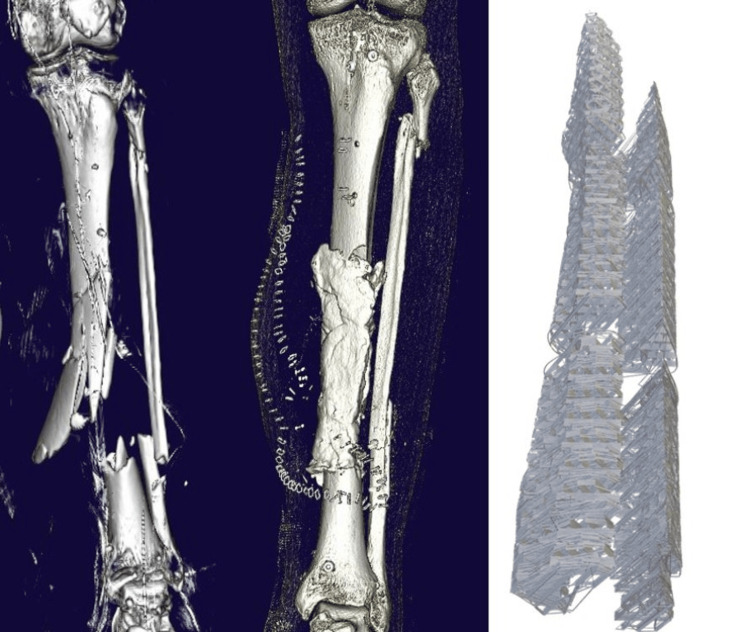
Three-dimensional reconstructions of the CT images of the initial injury and after the first stage IMT, and the virtually designed cage. IMT: Induced membrane procedure
